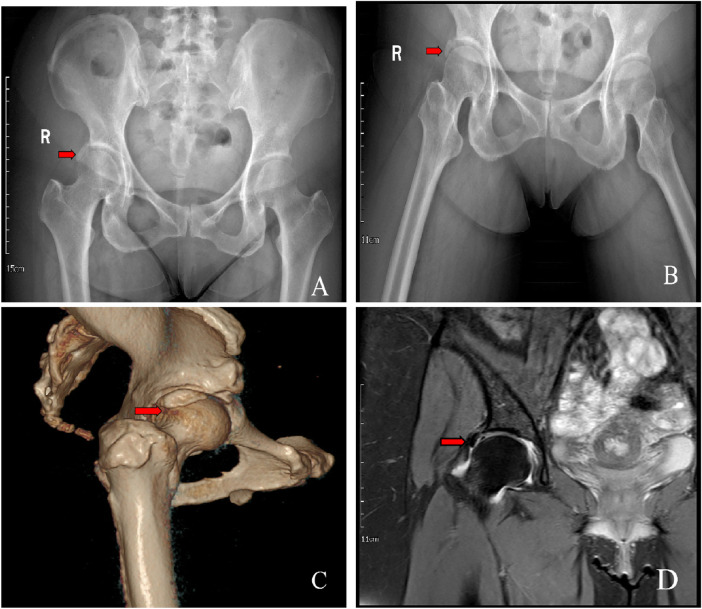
Preoperative radiographs [(A) anteroposterior view; (B) dunn view] and three-dimensional CT [(C) reconstructed view] demonstrate a high-density shadow at the anterolateral edge of the right acetabulum. Preoperative MRI [(D) Coronal view] shows right acetabular labrum calcification and injury.
